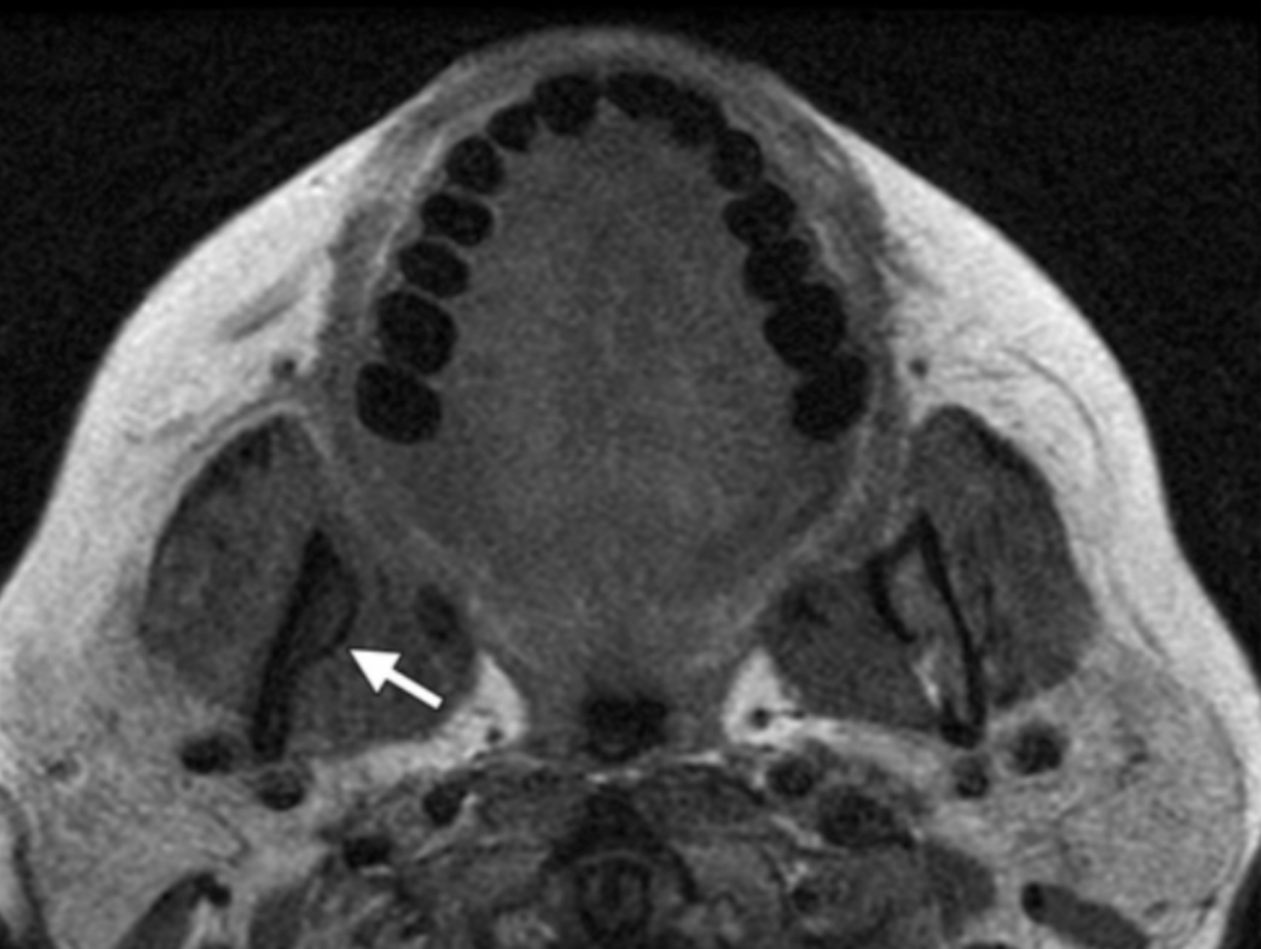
The appearance of ONJ on MRI modality: T1-weighted image The image shows reduced signal intensity in the right mandibular ramus (white arrow) ONJ: osteonecrosis of the jaw; MRI: magnetic resonance imaging
